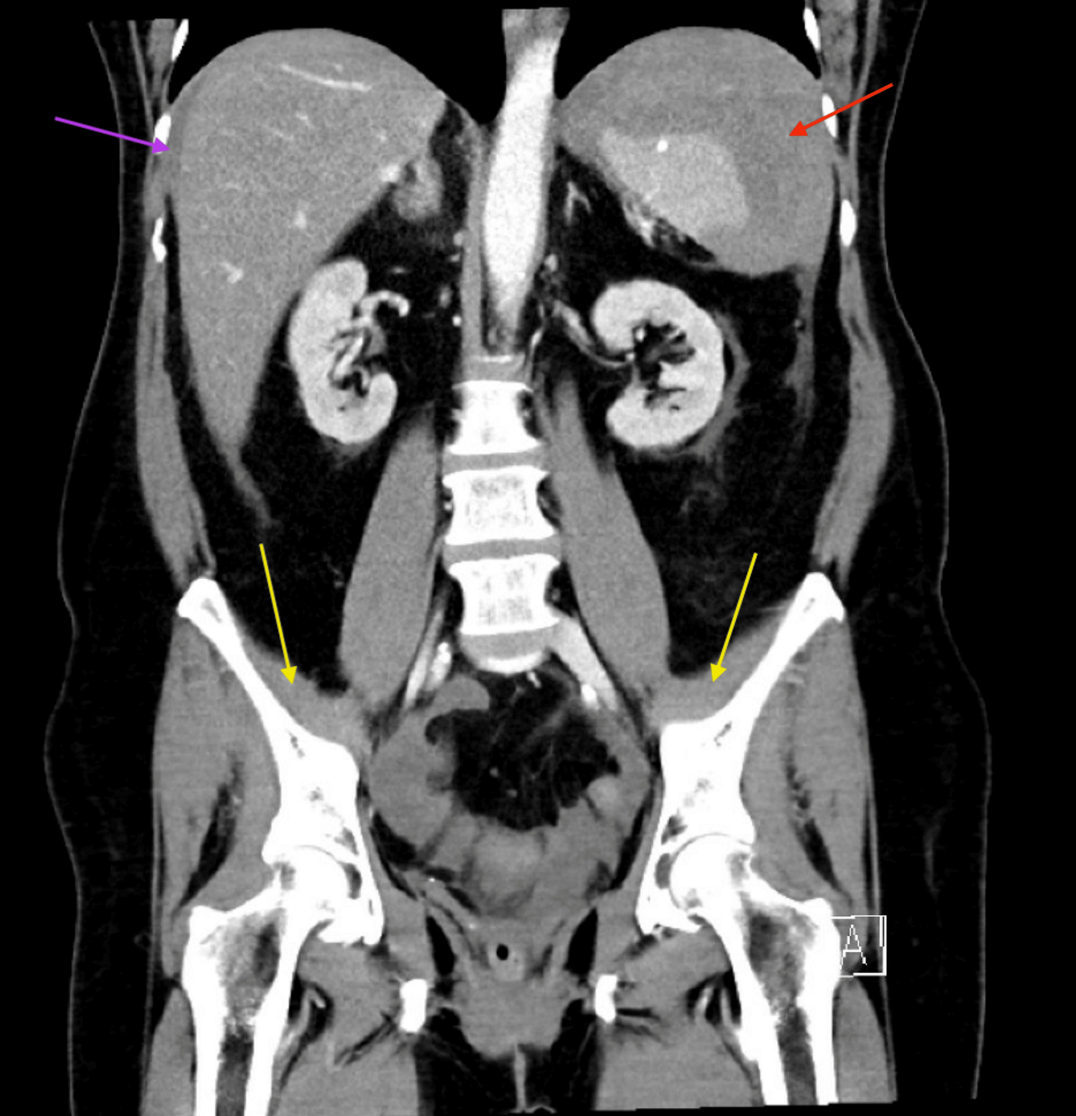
Computed tomography of the abdomen and pelvis with intravenous contrast Coronal view demonstrates a large perisplenic hematoma (red arrow) with small-to-moderate volume hemoperitoneum (yellow arrows) and blood surrounding the liver (purple arrow), without evidence of free air to suggest a bowel perforation. These findings were consistent with splenic injury, a rare but documented complication following colonoscopy.
